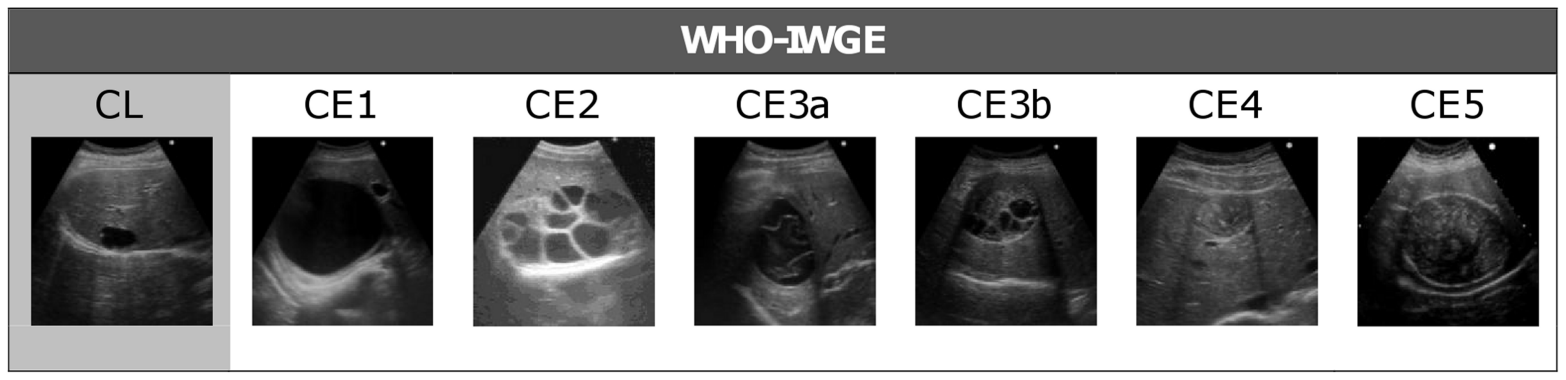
WHO-IWGE ultrasound classification of echinococcal cysts. CE1 and CE2 (active cysts), CE3A and CE3B (transitional cysts), and CE4 and CE5 (inactive cysts) [8].
